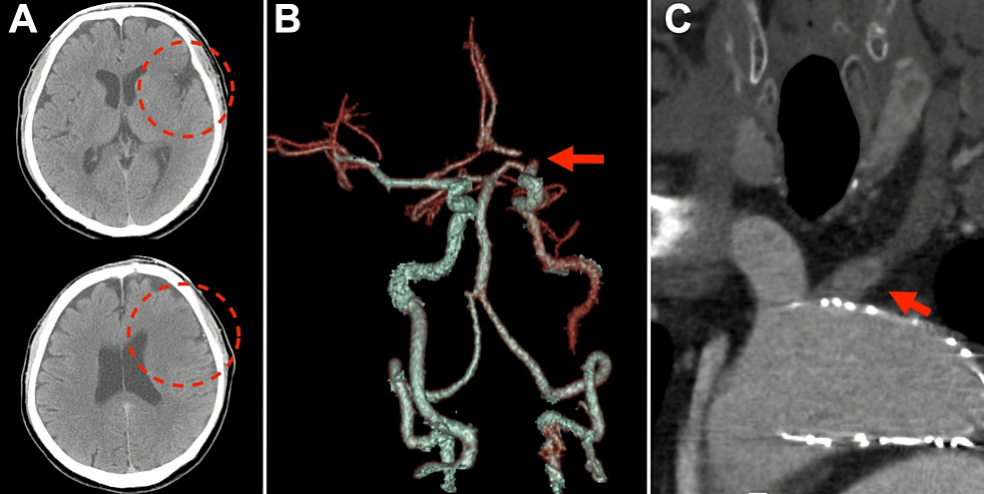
Computed tomography and computed tomography angiography on admission. Computed tomography shows an acute ischemic stroke in the left middle cerebral artery area. The upper one is at the level of the basal ganglia, and the lower one is at the level of the lateral ventricles (A). Computed tomography angiography (CTA) shows tandem occlusion of the proximal left common carotid artery (CCA) and terminal portion of the left internal carotid artery (B). The arrow indicates the occlusion of the proximal left CCA in the coronal section (C).
